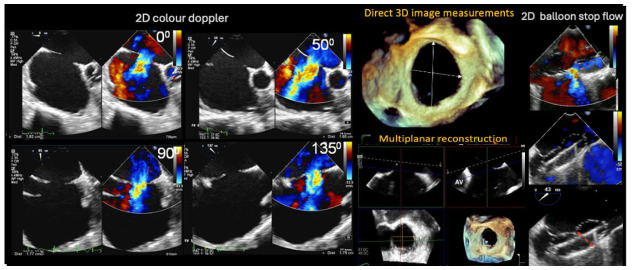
Methods for secundum atrial septal defect (ASD) device sizing. From left to right: 2D colour measured in the four standard planes with colour Doppler; largest ASD dimension in cardiac cycle (around end ventricular systole); measure from edge to edge of the 2D colour flow; the formula used to calculate the device size is given in Table 2. The second method uses 3DE imaging, 3D live zoom mode (see Table 1) acquisition and either a direct measure in still frame where ASD appears largest (long and short axis dimensions) or using multiplanar reconstruction (see Table 2). Lastly using 2D echo colour Doppler placed over the ASD/septum, a balloon is gradually inflated and colour flow is monitored until the flow stops (no longer seen within edges of balloon and defect is fully occluded); this method will ensure septum is not overstretched and ASD device oversized.
